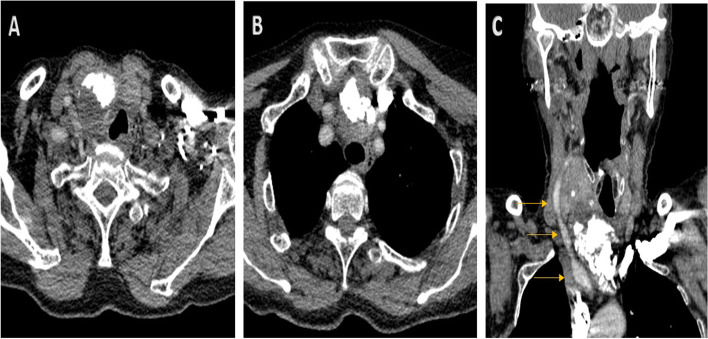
Post-contrast neck and upper chest CT axial (A, B) and coronal images (C). Heterogenous enhancing right thyroid lobe soft tissue mass with coarse calcification is seen displacing the airway to the contralateral side and extending down to the superior mediastinum abutting the aortic arch branches with clear fat plane in-between. It was seen also contacting the right CCA along its length without vascular invasion (arrows). No pathological cervical LNs
